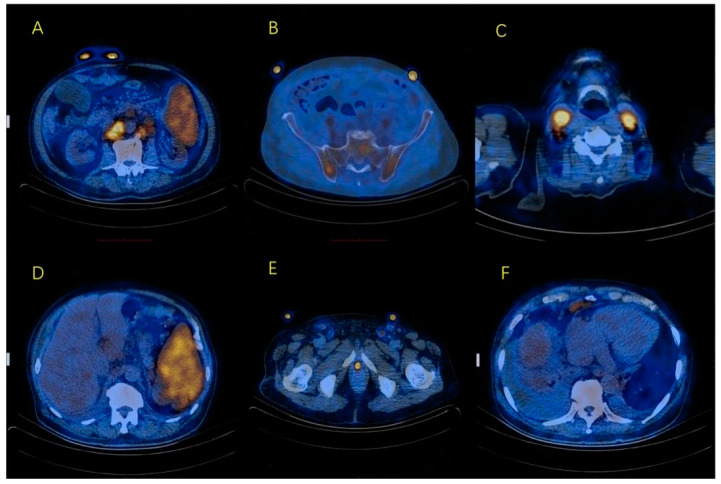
PET/CT showed enlargement of multiple lymph nodes with increased glucose metabolism. (A) enlarged retroperitoneal lymph nodes; (B) slightly increased glucose metabolism of the pelvis with no bone destruction; (C) enlarged cervical lymph nodes; (D) splenomegaly with increased glucose metabolism; (E) enlargement of bilateral inguinal lymph nodes; (F) enlarged lymph nodes in cardiophrenic angle.
